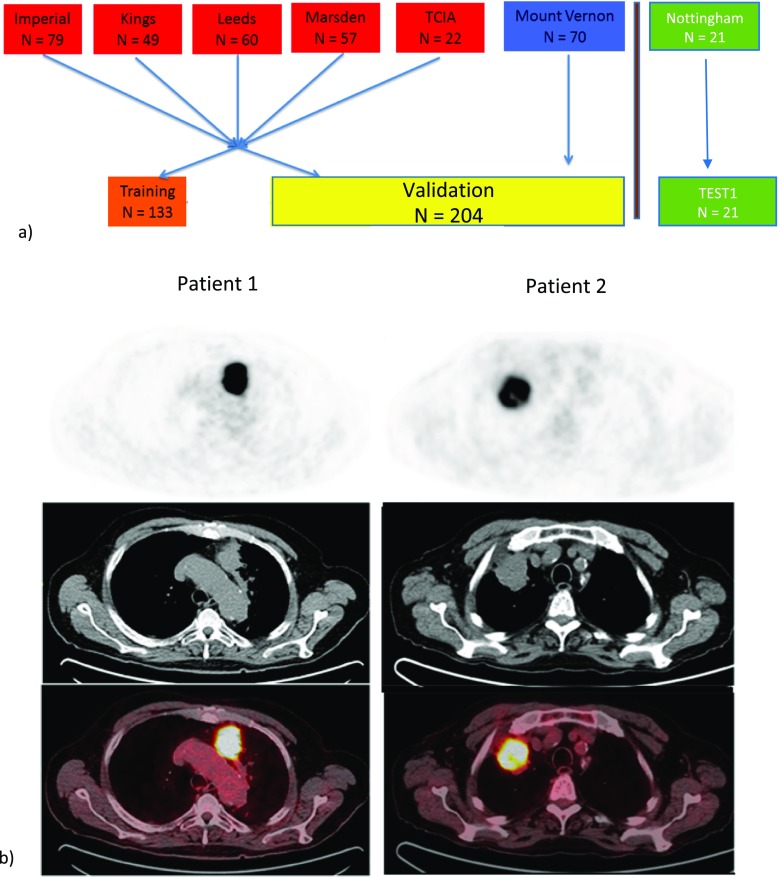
Overview of centers and PET images.a Overview of the centers contributing to the study and how the data were randomly divided into training, validation or independent test set. TCIA, The Cancer Imaging Archive. b Typical images from the PET/CT scans of two patients including PET, CT, and fusion images. Patient 1 (age 74, squamous cell carcinoma, stage IIA, tumour volume 22.6, overall survival 8 months) with the lower stage and smaller volume primary lesion had a worse survival outcome than patient 2 (age 77, squamous cell carcinoma, stage IIIA, tumour volume 26.5, overall survival 33 months) with the higher tumour stage
